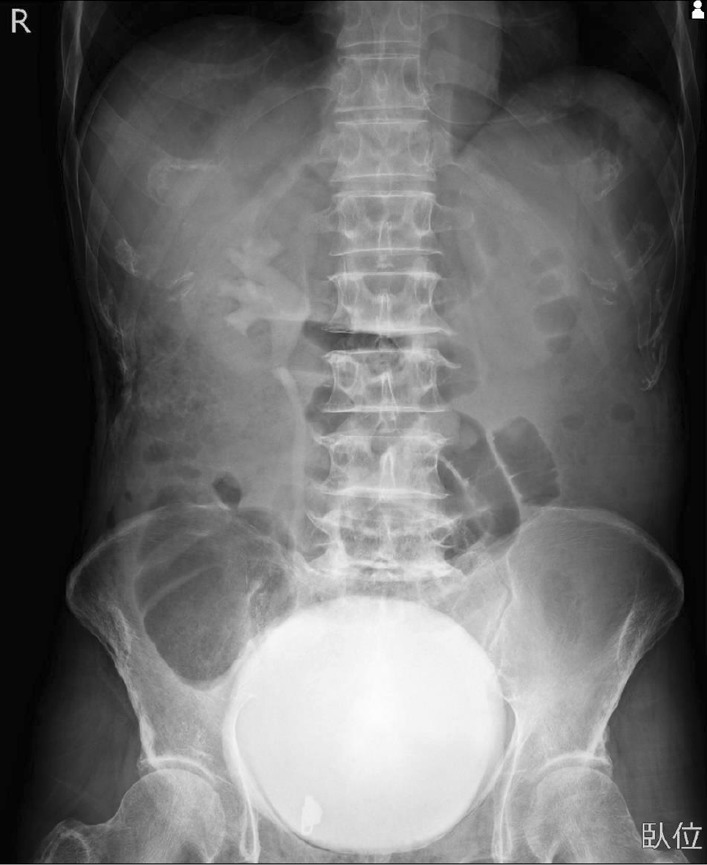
X-ray examination results 20 h after admission revealed residual radiopaque dye used for computed tomography (CT) in the dilated bladder
